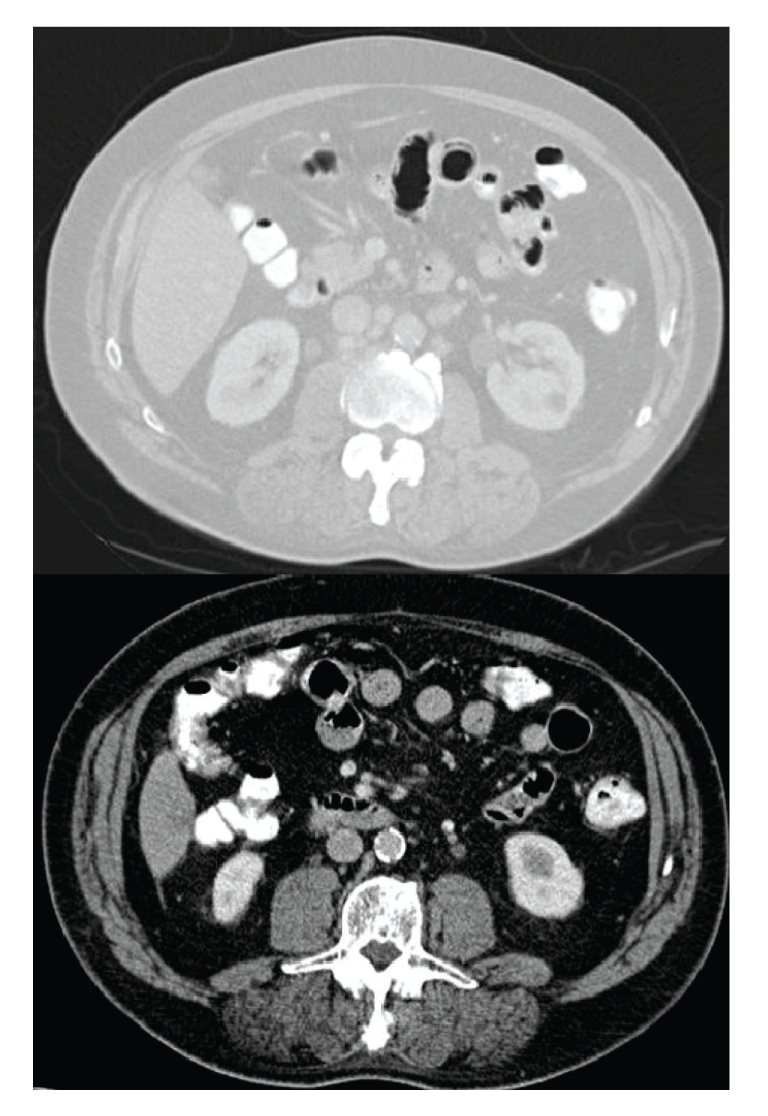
Above: CT scan June 2010 revealing abdominal para-aortic lymphadenopathy. Below: unremarkable CT scan, November 2009.
